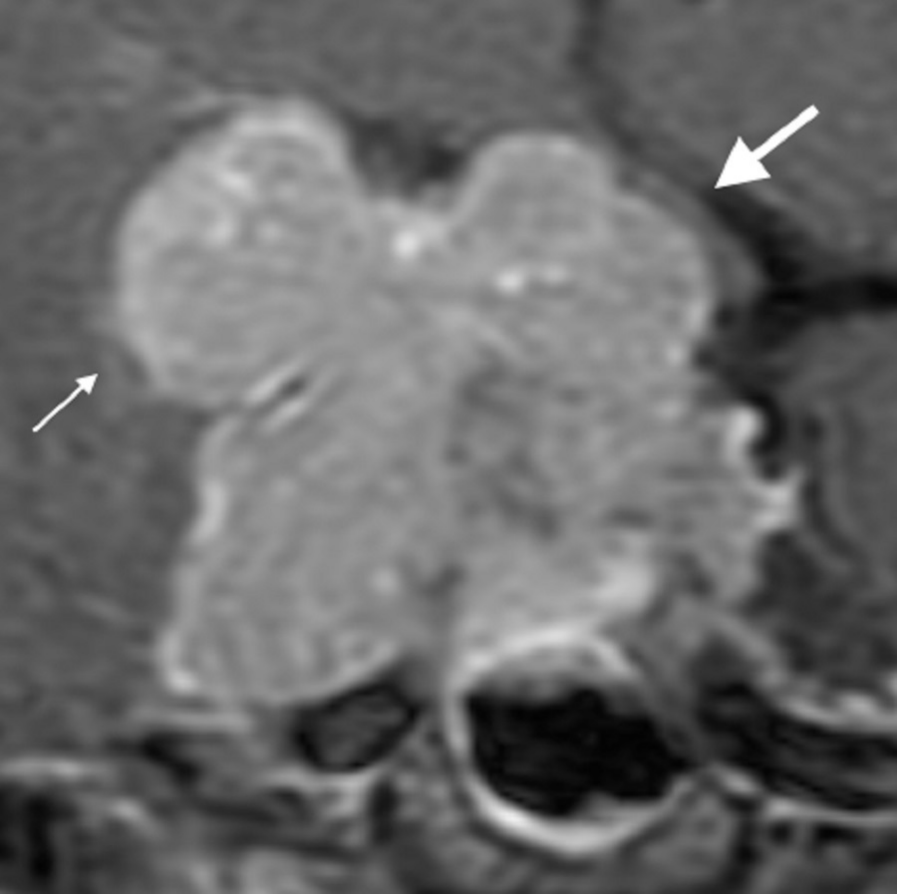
Coronal Post-Contrast MRI Sequences Prior to craniotomy, demonstrating marked expansion with suprasellar extension and compression of optic chiasm (arrow) and intracranial invasion into right middle fossa
